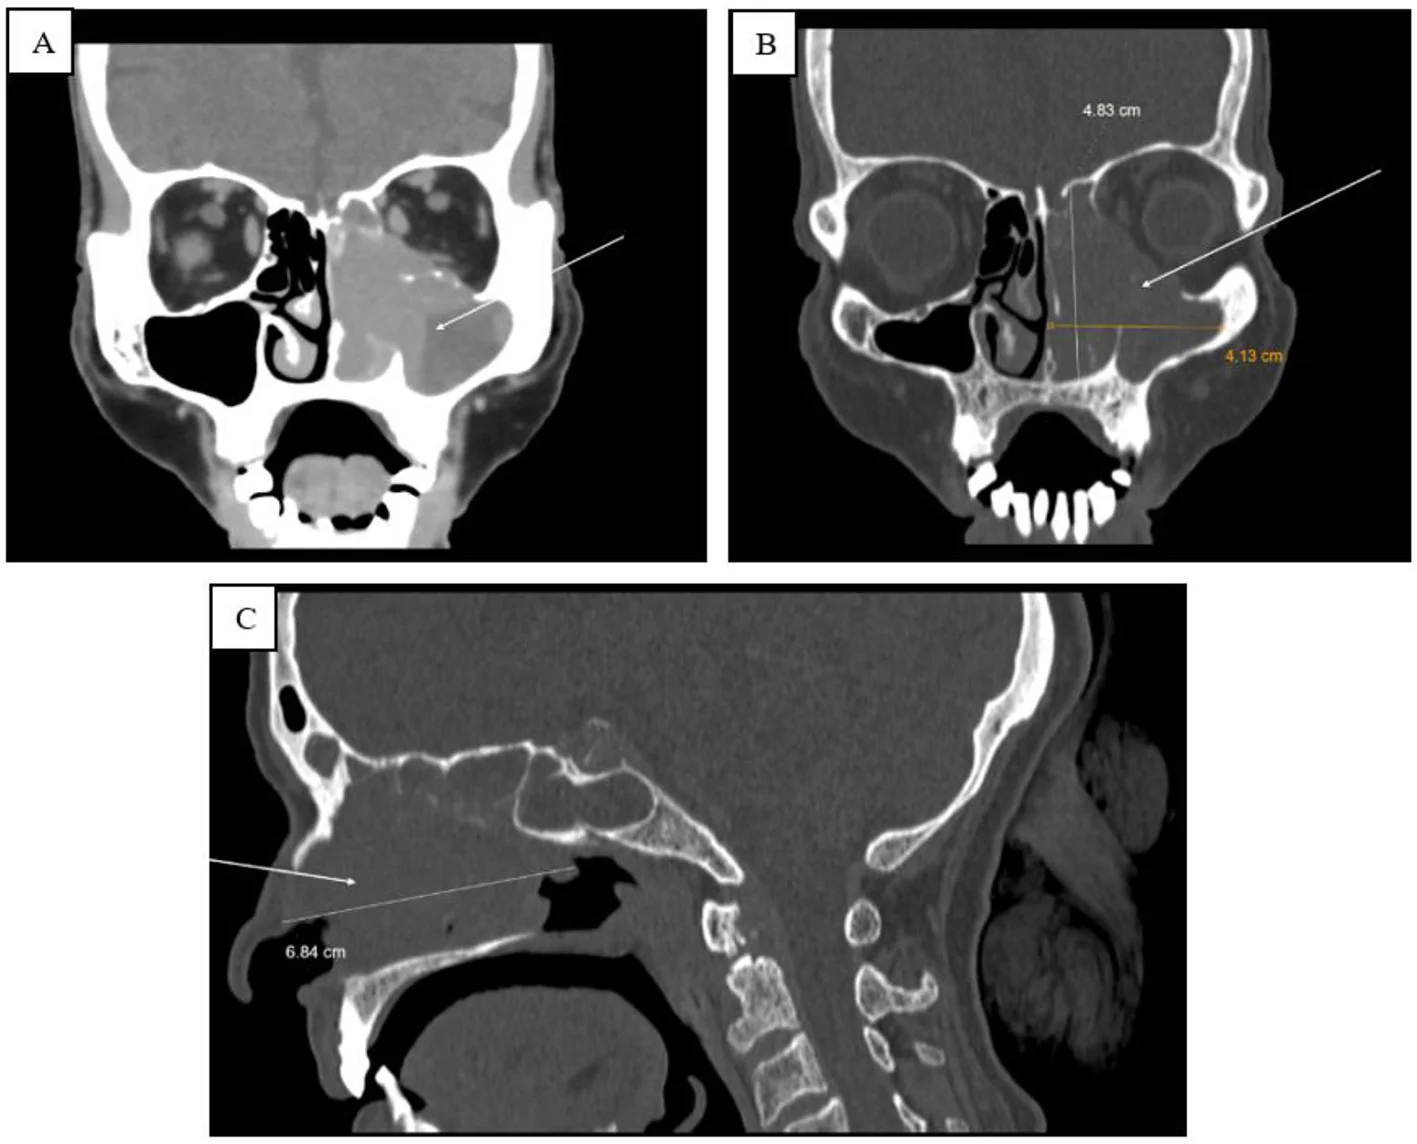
Contrast-enhanced computed tomography of the paranasal sinuses. (A) Coronal soft-tissue window. (B) Coronal bone window. The coronal images show a heterogeneously enhancing soft-tissue lesion occupying the left maxillary sinus, with extension into the left nasal cavity and adjacent sinuses. (C) Sagittal bone window showing the craniocaudal extent and posterior sinonasal involvement. Bone thinning/destruction and erosion of the lamina papyracea were noted radiologically.
